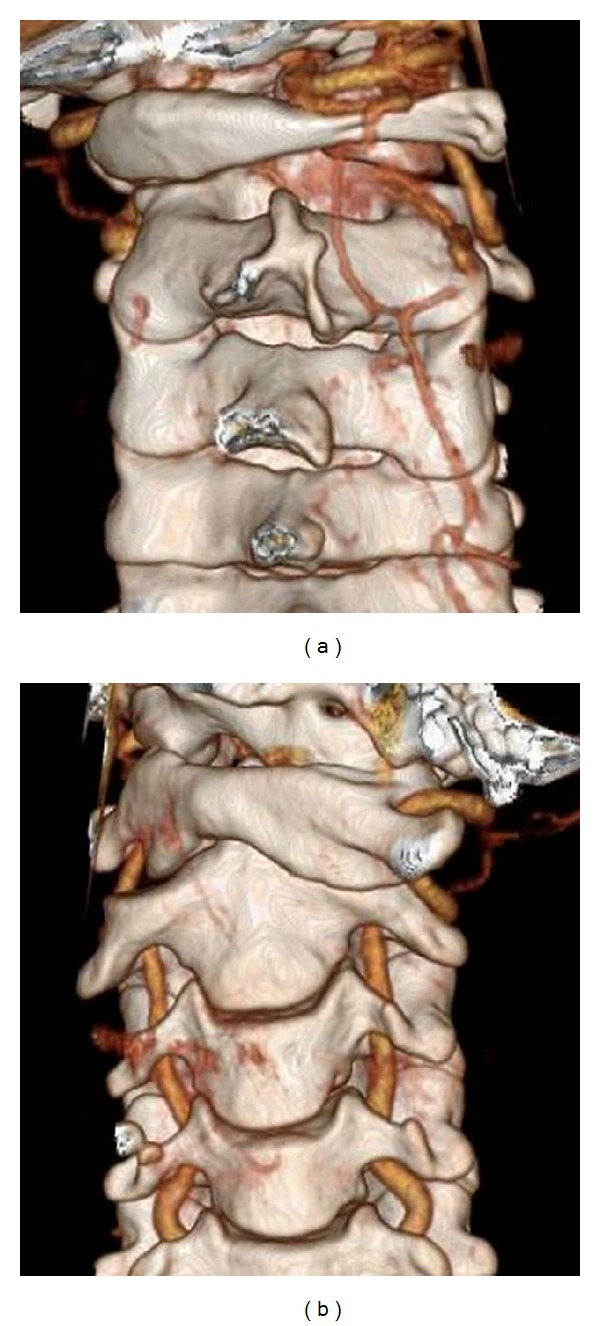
The three-dimensional CT showed atlantoaxial rotatory fixation of Fielding classification type I. (a) Posterior view and (b) anterior view.
